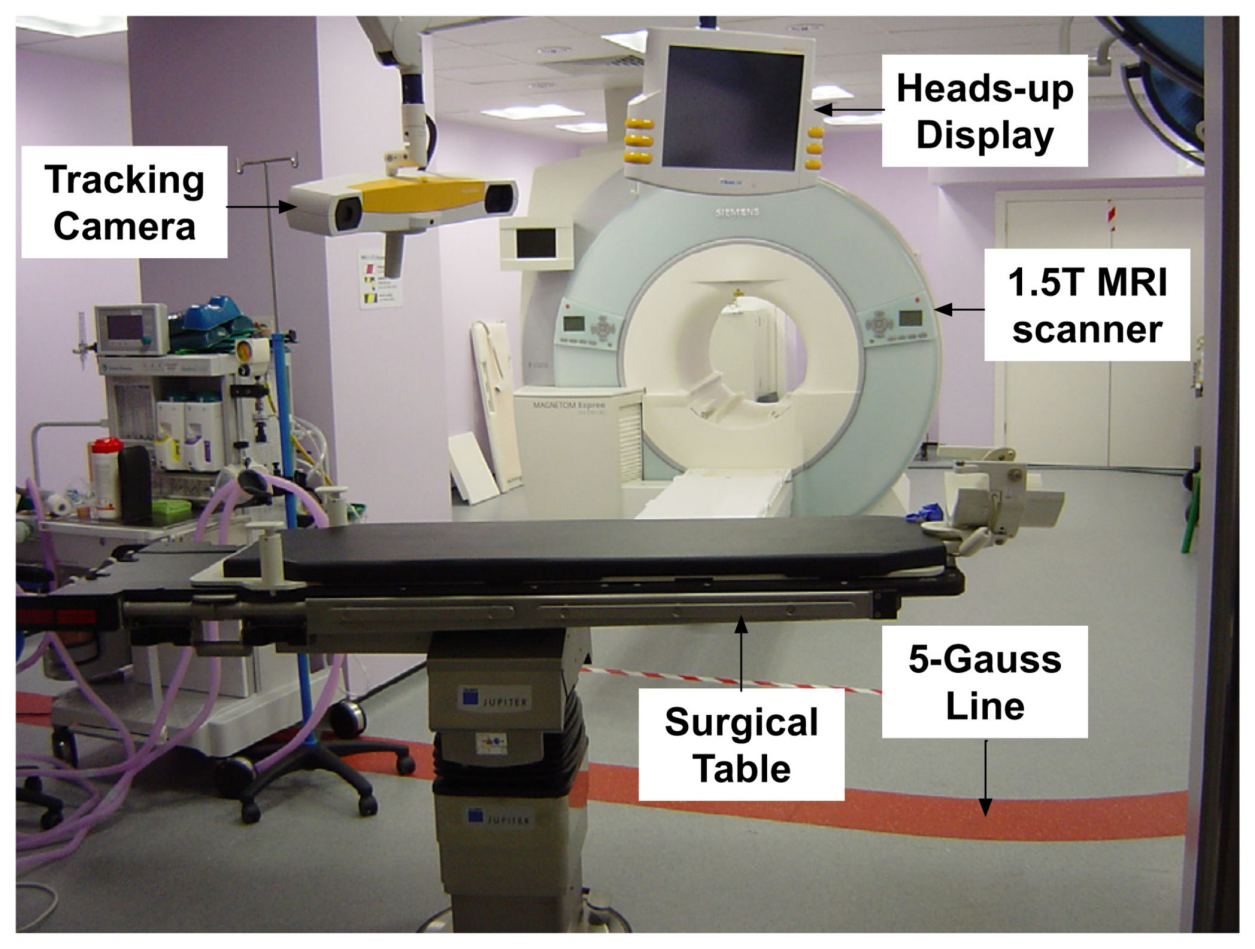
Interventional MRI surgical suite at the National Hospital for Neurology and Neurosurgery with a 1.5 T MR scanner and neuronavigation equipment. The surgical table interfaces with the scanner to enable the patient to be moved in and out of the scanner efficiently during surgery.
